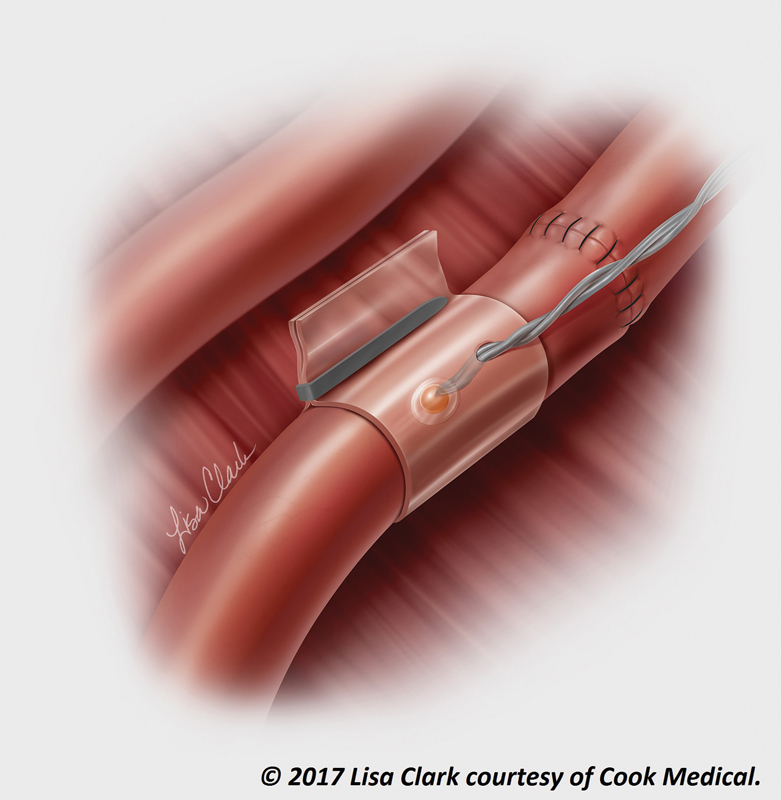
Cook–Swartz Doppler probe.
